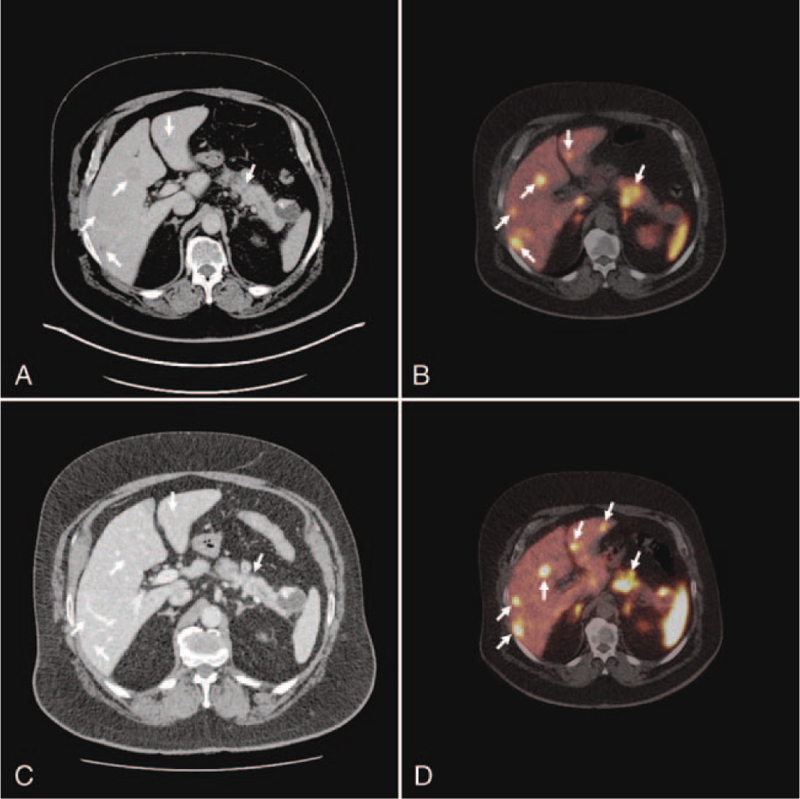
Initial and follow-up imaging of Case 2. (A) The initial CT detected a discrete hyperdense lesion in the pancreatic corpus/tail and hyperperfused areas in both liver lobes (white arrows). (B) The initial Ga-68-DOTANOC PET/CT revealed a large SSR-positive lesion within the pancreatic corpus/tail and multiple SSR-positive lesions within both lobes of the liver (white arrows). (C) The follow-up CT imaging demonstrated partial remission of the hyperdense lesion within the pancreatic corpus/tail and remission of the hepatic metastasis (white arrows). (D) The follow-up Ga-68-DOTANOC PET/CT showed progressive SSR-positive hepatic metastasis in both liver lobes. CT = computed tomography, PET/CT = positron emission tomography/computer tomography, SSR = somatostatin receptor.
